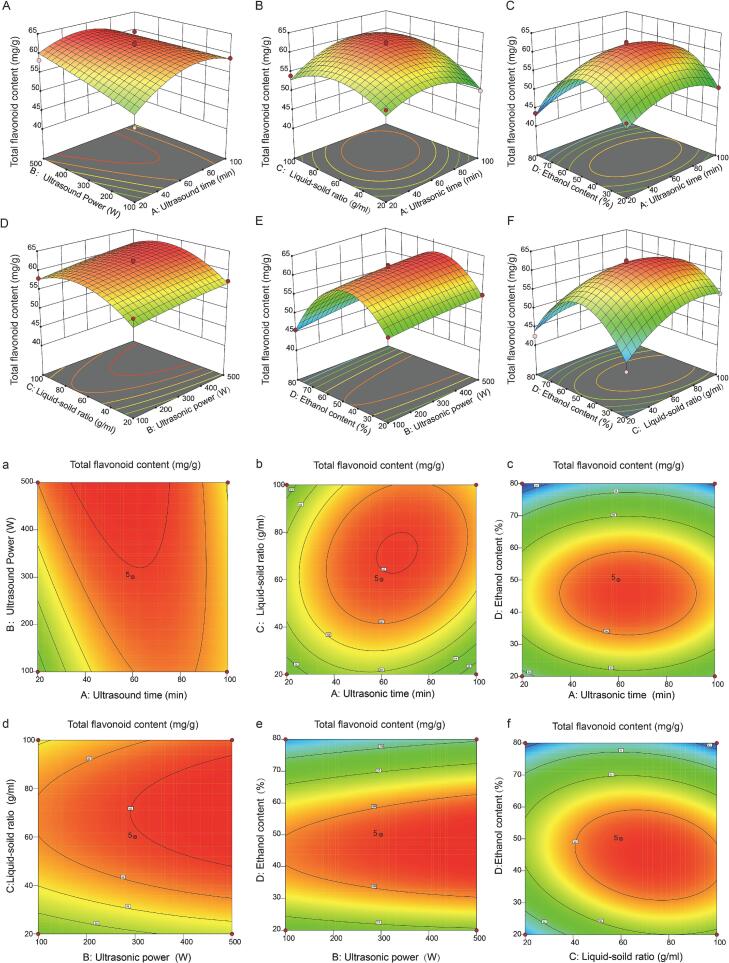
Response surface and contour plots showing the interactive effects of UAE variables on total flavonoid content in *A. spinulosa* leaves. (A, a) Ultrasound time and ultrasound power. (B, b) Liquid-to-solid ratio and ultrasound time. (C, c) Ethanol concentration and liquid-to-solid ratio. (D, d) Liquid-to-solid ratio and ultrasound power. (E, e) Ethanol concentration and ultrasound power. (F, f) Ethanol concentration and liquid-to-solid ratio.
